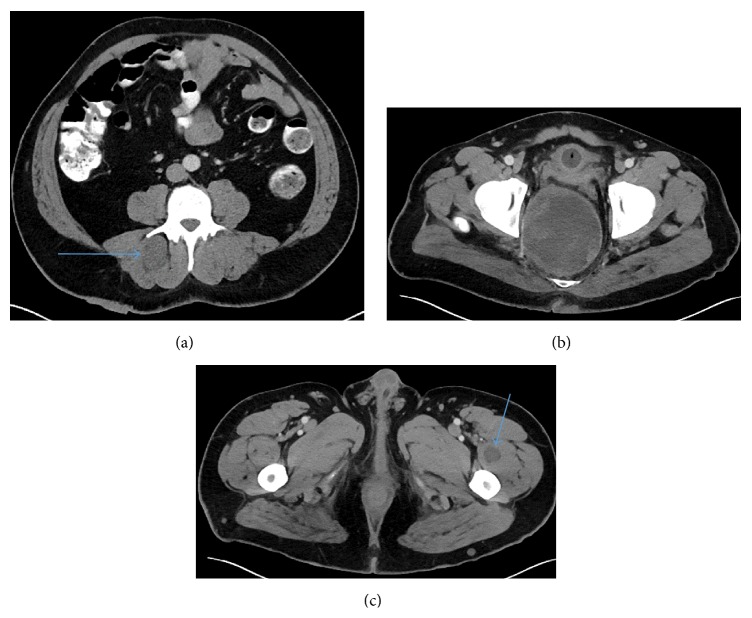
(a) Axial CT right shows right erector spinae/multifidus muscle neurofibroma (arrow); the attenuation of the mass is about the same to slightly less than that of muscle. (b) Axial CT shows heterogeneous pelvic mass. (c) Axial CT shows left vastus medialis/intermedius muscle fibroma (arrow); the attenuation of the mass is less than that of muscle.
